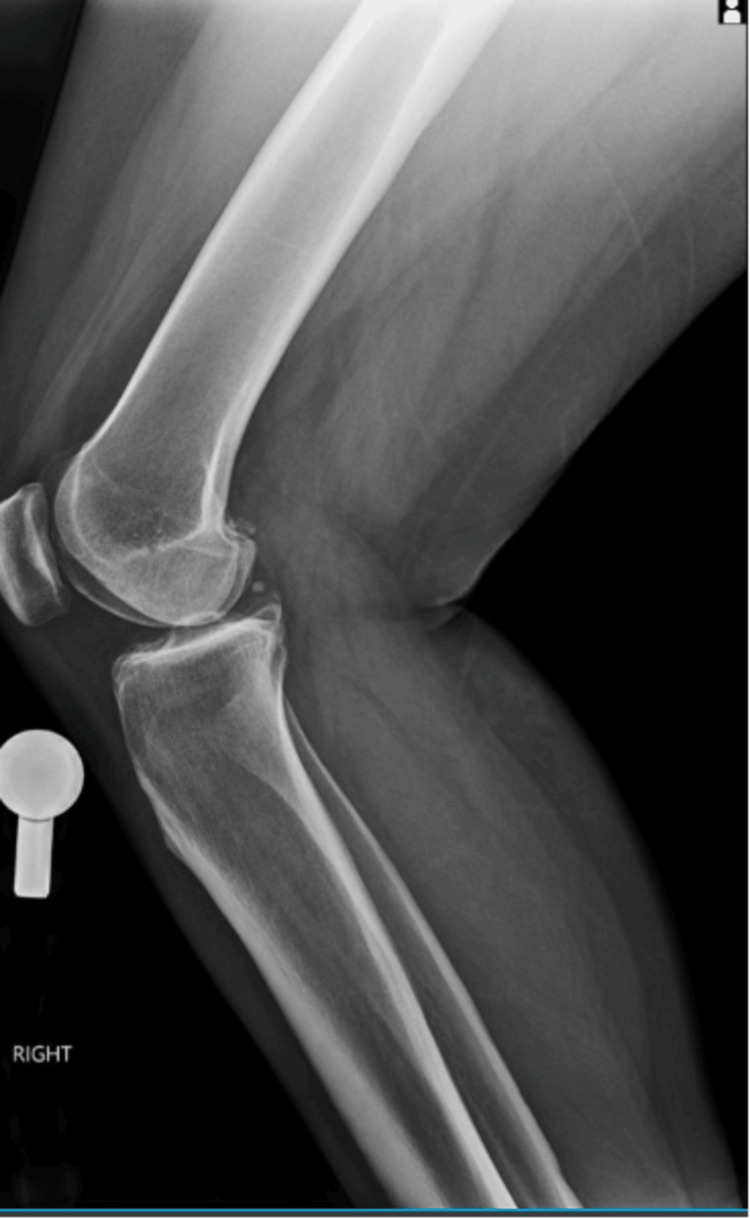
Lateral radiograph of the right knee demonstrating preserved sagittal alignment
